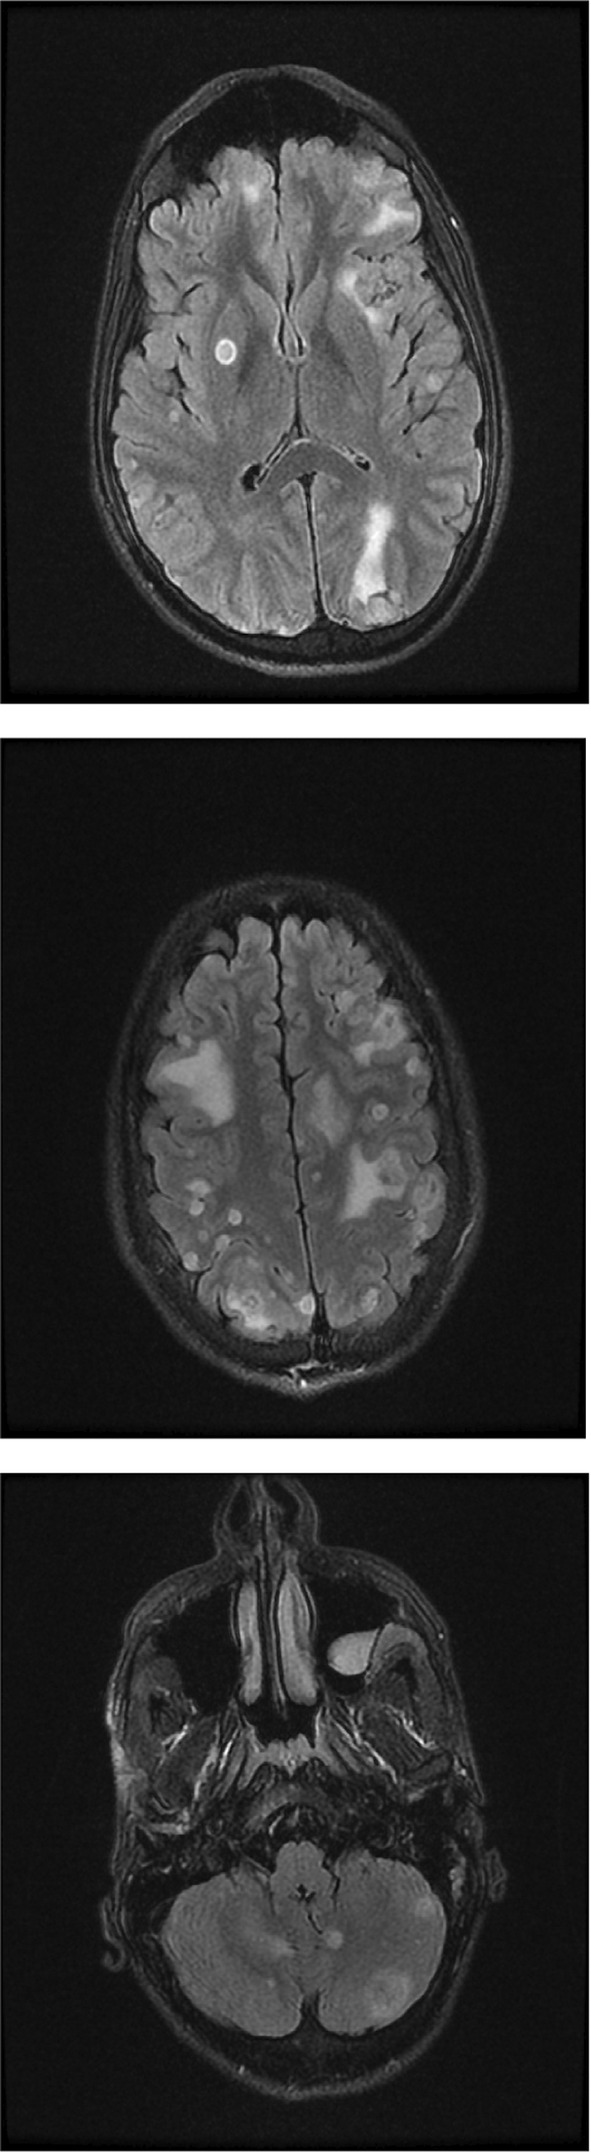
Patient's brain magnetic resonance image.
